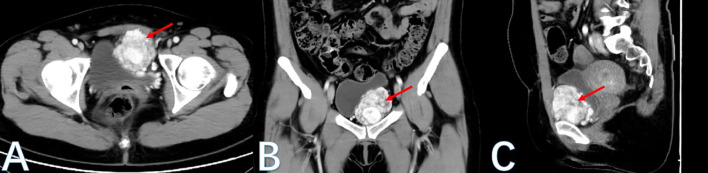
Computed tomography urography (CTU) images of the asymptomatic pelvic solitary fibrous tumor (SFT) in Case 3. (A) Axial contrast-enhanced CTU image showing a well-circumscribed, heterogeneous soft-tissue mass measuring 5.4 × 5.1 × 5.6 cm located anterosuperior to the bladder. (B) Coronal contrast-enhanced CTU image demonstrating marked heterogeneous enhancement during the arterial phase and multiple tortuous feeding vessels surrounding the lesion. (C) Sagittal contrast-enhanced CTU image confirming mild compression of the anterior bladder wall by the mass and clear interface with adjacent pelvic soft tissues. Red arrow indicates the pelvic SFT.
